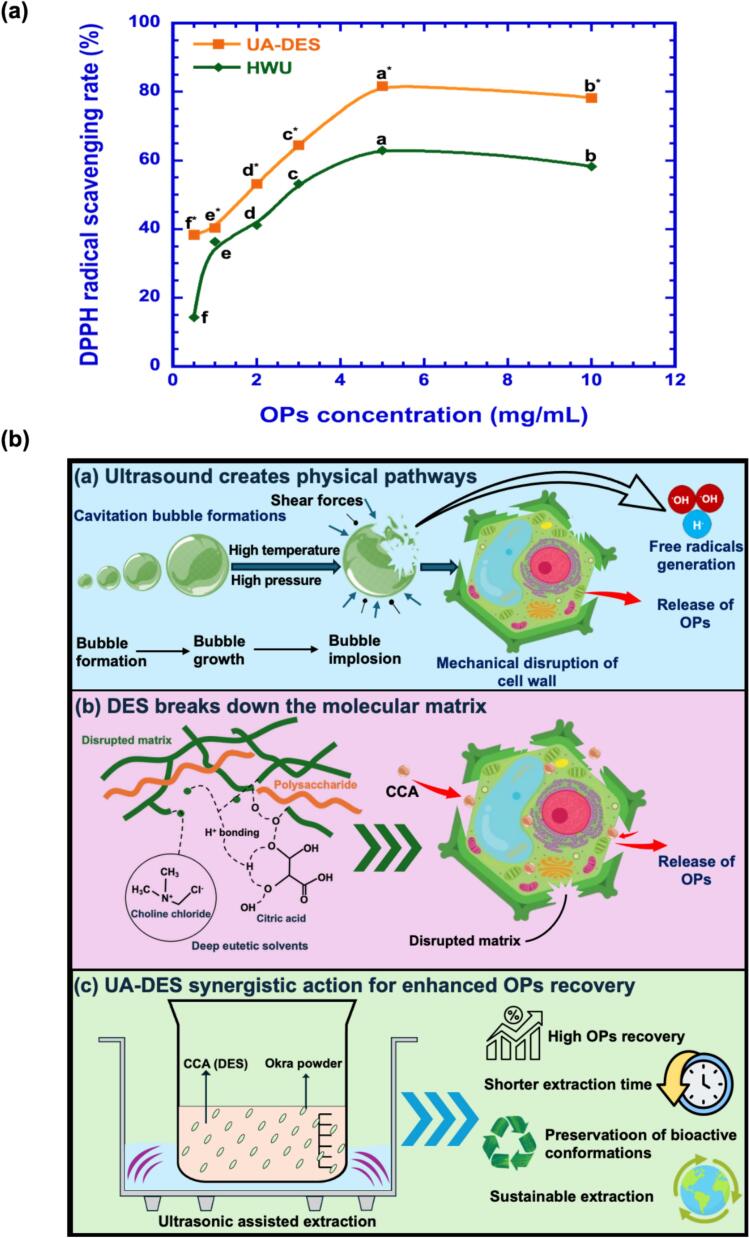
(a) DPPH radical scavenging activity of OPs obtained by UA-DES and HWU. OPs samples extracted by UA-DES and HWU were tested at concentrations ranging from 1 to 10 mg/mL. DPPH concertation was 0.1 mM. Data are expressed as mean ± SD (n = 3). Different letters above the data points indicate significant differences among concentrations within the same extraction method, as determined by one-way ANOVA followed by Tukey’s post hoc test. Statistical significance was considered at *P* < 0.05. (b) Proposed mechanisms of deep eutectic solvent coupled with ultrasound-assisted (UA-DES) extraction of OPs. In this system, choline chloride/citric acid-based DES penetrates the plant matrix and disrupts hydrogen-bonding networks, while ultrasound cavitation generates bubbles and shear forces that mechanically break cell walls. The combined effects facilitate solvent infiltration and promote the release of intracellular polysaccharides and phytochemicals. The citric acid component contributes to pH modulation and chelation of metal ions, thereby enhancing polysaccharide solubilization and extraction efficiency. Additionally, free radical generation during cavitation may further increase solvent penetration and extraction performance.
